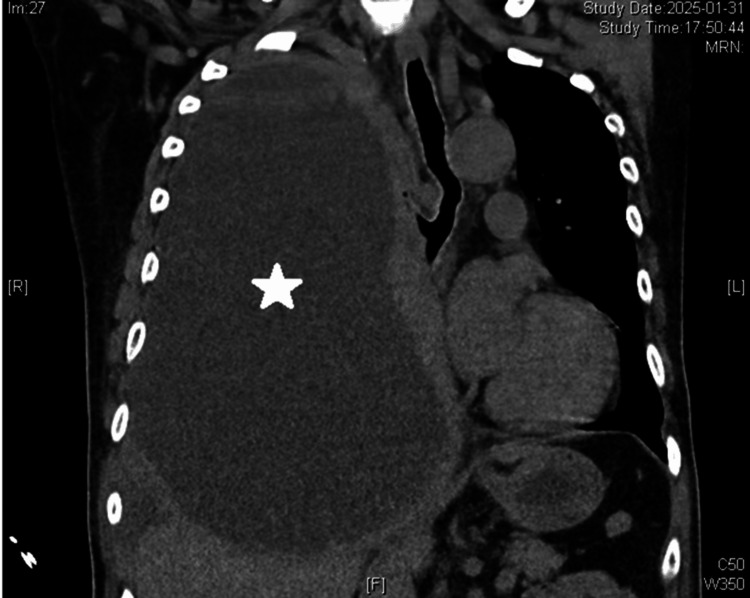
Admission Chest CT Scan Coronal chest images demonstrate a large right-sided pyopneumothorax (asterisk). The mediastinal window confirms extensive pleural effusion.
